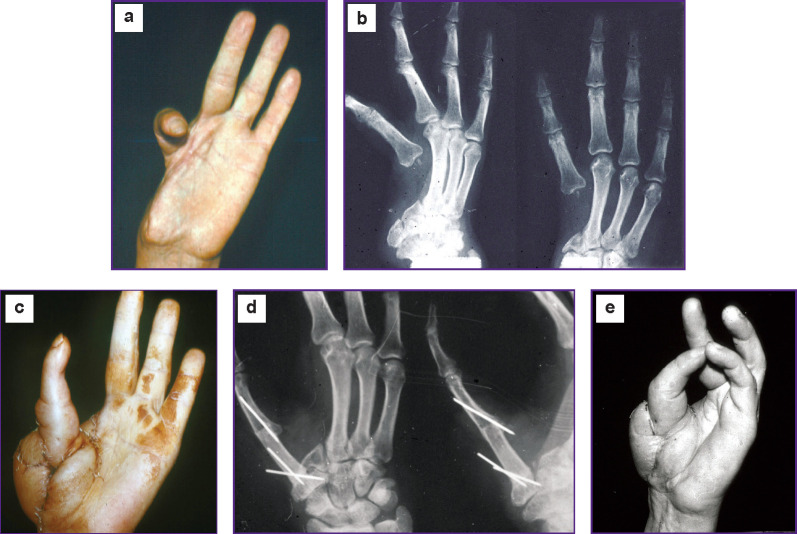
Treatment outcome in patient S., 43 years old, with a defect in the radial edge of the hand one year after transplantation: (a) the hand appearance before surgery; (b) X-ray images of the hand before surgery; (c) the hand appearance after plastic surgery on its radial edge, an accustomed sentinel flap can be seen; (d) X-ray images of the hand one year after repositioning the second finger and transplanting a fragment from the second metatarsal bone; a bone marrow canal and complete consolidation can be identified; (e) the opposing function of the first finger
